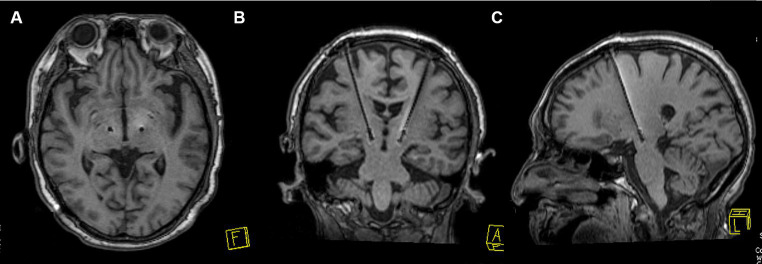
STN-DBS electrode position verification image. (A–C) An MRI example of electrode placement in the STN is shown to verify the accuracy of electrode placement.
